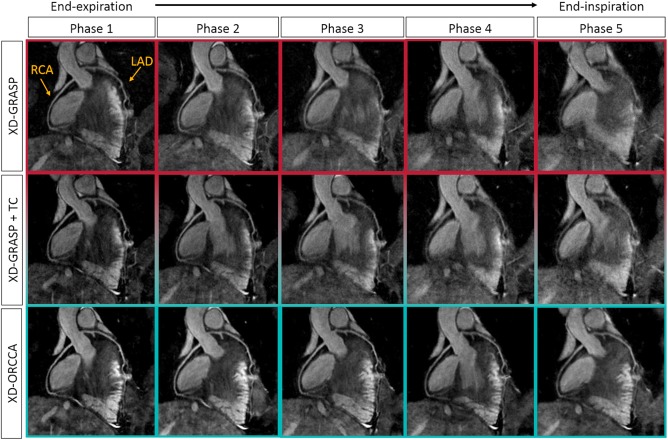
Reformatted respiratory‐resolved images obtained for 1 representative subject using XD‐GRASP (eXtra‐Dimensional Golden‐angle RAdial Sparse Parallel MRI; top), XD‐GRASP with intrabin motion correction (translational correction [TC]; middle), and the proposed XD‐ORCCA (Optimized Respiratory‐resolved Cartesian Coronary MR Angiography; bottom). For each method, 5 respiratory phases are shown: from end‐expiration (left) to end‐inspiration (right). Each image shows the right coronary artery (RCA) and the left anterior descending coronary artery (LAD). Including TC in XD‐GRASP slightly improved the quality of the respiratory‐resolved images. However, motion artifacts are still visible in phases 4 and 5. The XD‐ORCCA method considerably improves the vessel visibility and sharpness of both coronary arteries. Nevertheless, XD‐GRASP provides good‐quality end‐expiration images, which usually contain less respiratory motion. However, the coronary vasculature cannot be clearly visualized in near end‐inspiration phases
